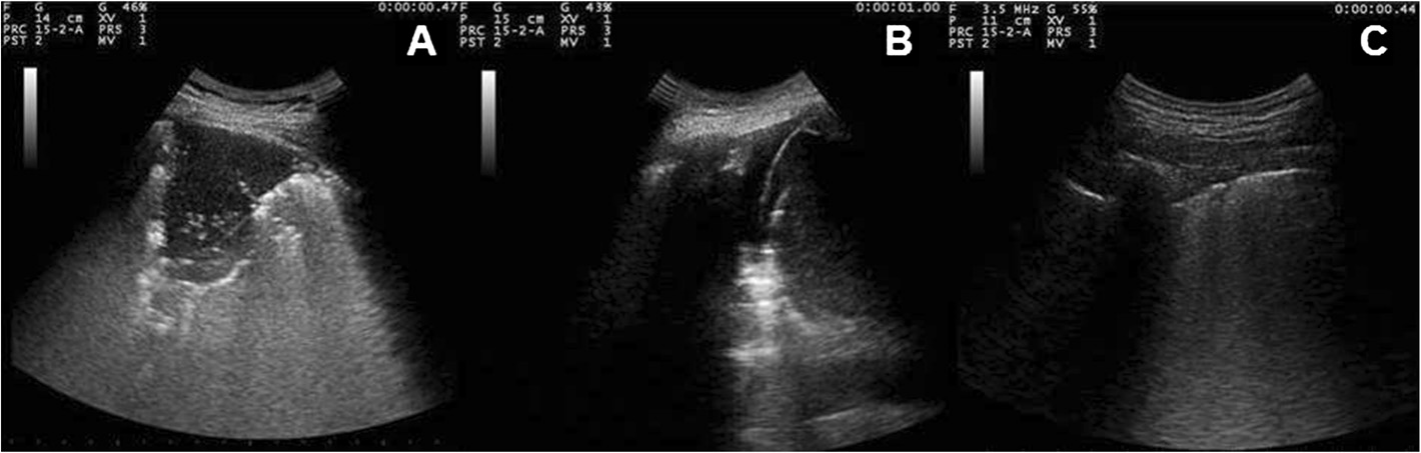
Chest ultrasonographic patterns: lung consolidation (A), pleural effusion (B) and sonographic interstitial syndrome (C).
